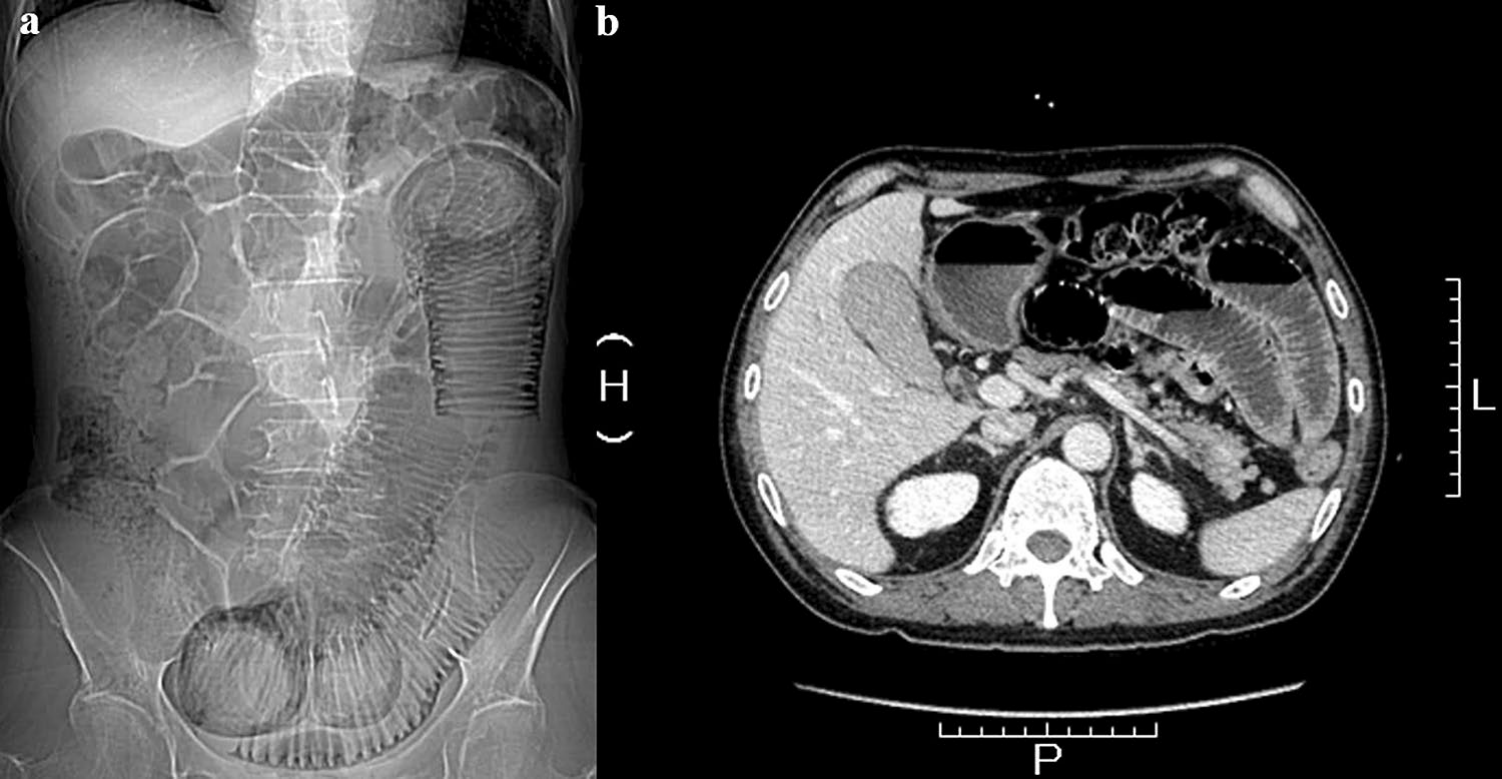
Typical radiological images of chronic intestinal pseudo-obstruction including plain radiograph (a) and computed tomography (b).
